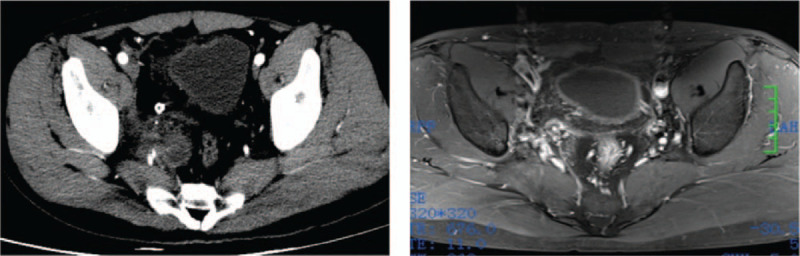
CT scan of the pelvis showing changes after cystectomy; a little cystic duct low-density shadow remains. MRI scan of the pelvis showing no recurrence of cysts after 3 months of surgical treatment.
